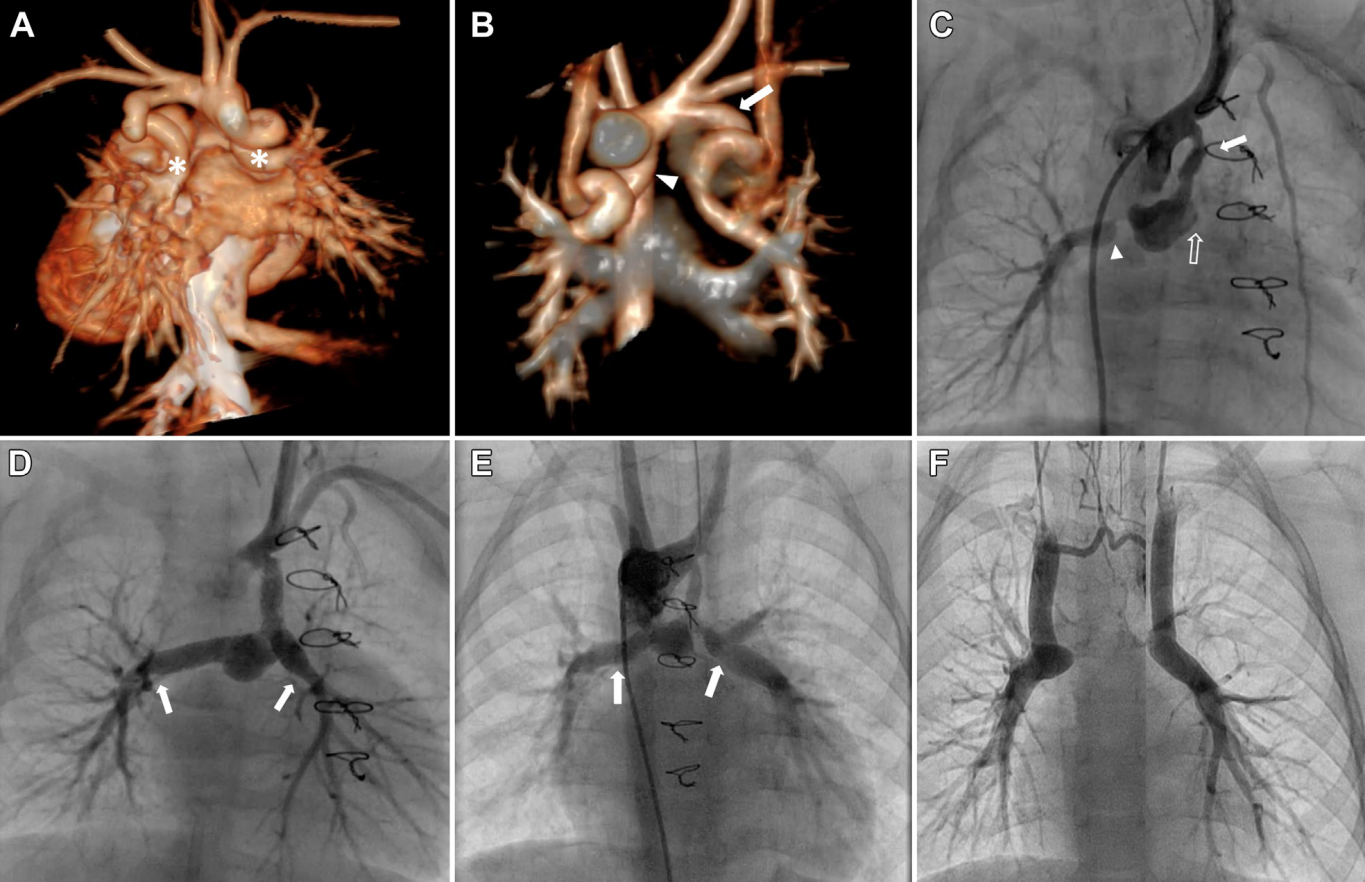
Transcatheter Resolution of a Failed Neoconfluence in Discontinuous Pulmonary Arteries (A, B) Three-dimensional volume-rendered computed tomography angiography shows bilateral ductal origin of both pulmonary arteries (asterisks). Right ductus arteriosus arising from the aortic isthmus (arrowhead) and left ductus arteriosus arising from the left brachiocephalic artery (arrow). (C) Angiography of a right aortic arch shows multiple-site obstruction: junction with right pulmonary artery (arrowhead) and left pulmonary artery (hollow arrow) and proximal portion of systemic-to-pulmonary shunt (white arrow). (D) Angiography after stent placement shows adequate flow in the systemic-to-pulmonary shunt; however, stenosis is evident in the distal right pulmonary artery and distal left pulmonary artery (white arrows). (E) Angiography before Glenn procedure shows the distal portions of both stents (white arrows) at a distance from pulmonary artery branching, as well as significant stenosis of the proximal left pulmonary artery. (F) Patent bilateral Glenn shunts with adequate caliber of distal pulmonary arteries, stents medially alongside the re-stenosed neoconfluence. Abnormal filling of the left upper pulmonary artery is also observed.
